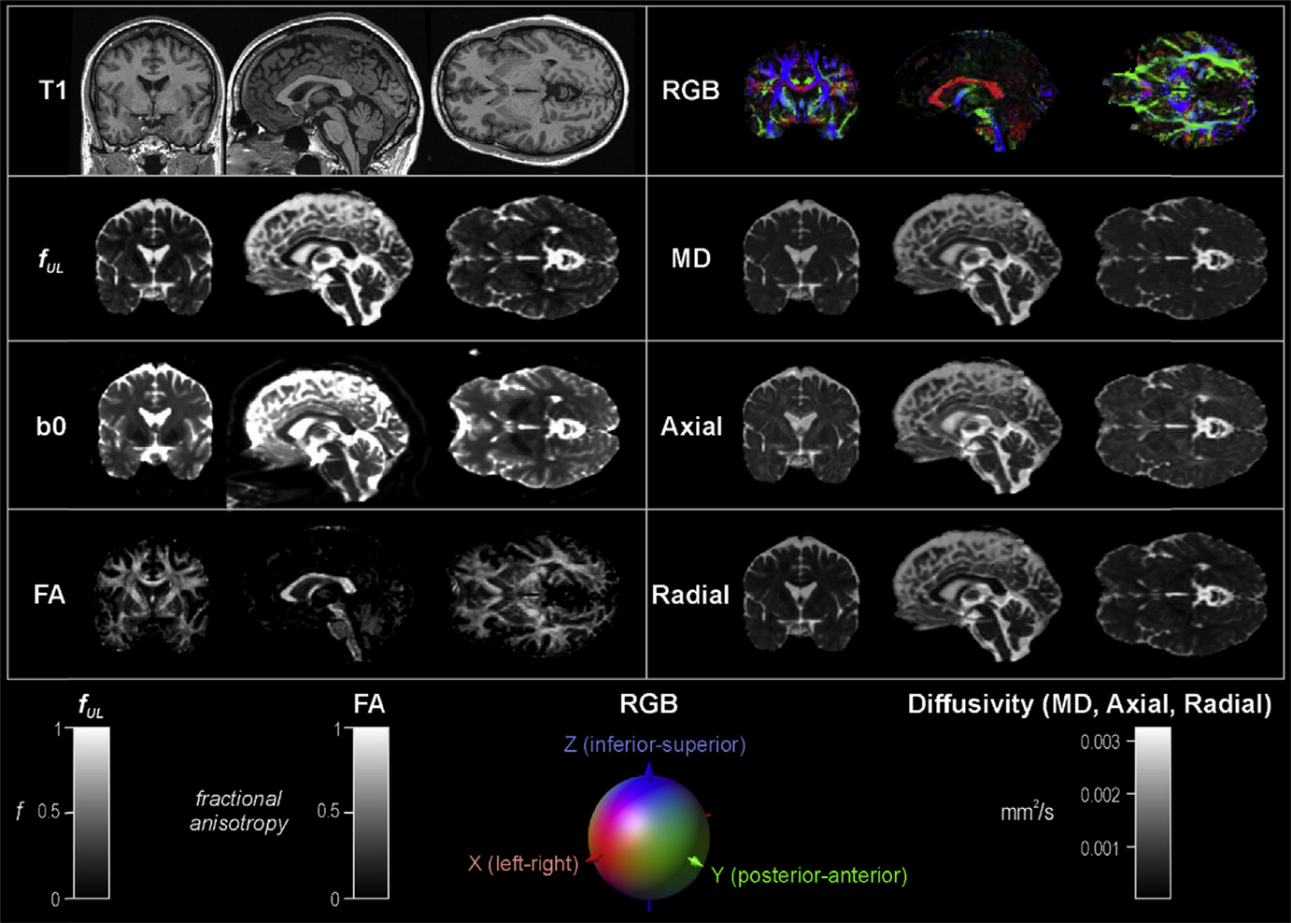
Example of DTI-based anatomy and indices for a single subject. T1: T1-weighted anatomical reference; all DTI-based images are in T1 space. *f*_*UL*_: free water fraction upper limit. b0: b0 image. FA: fractional anisotropy. RGB: color-coded directional anatomy, as per scale. MD: mean diffusivity. Axial: axial diffusivity. Radial: radial diffusivity. Scales for indices are at bottom.
